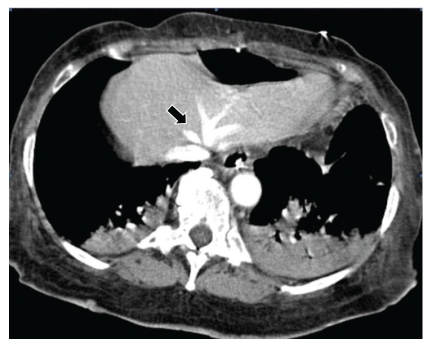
CT image of the liver shows normal contrast enhancement of hepatic venous system (*arrow*). It also shows bibasilar atelectasis.
